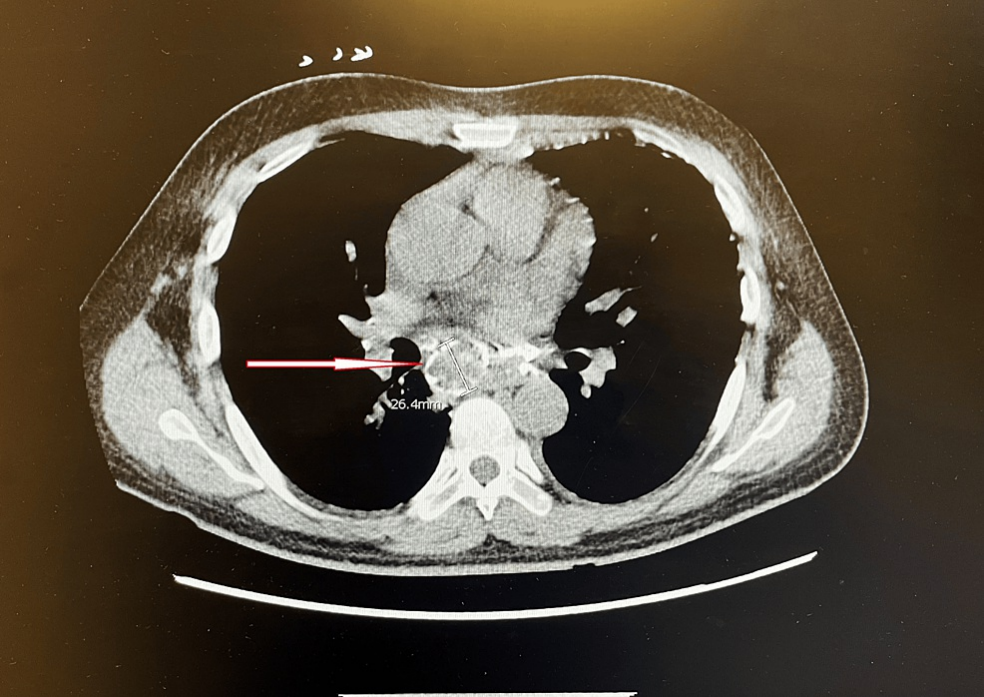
Axial computed tomography (CT) scan showing eggshell calcification (red arrow).
